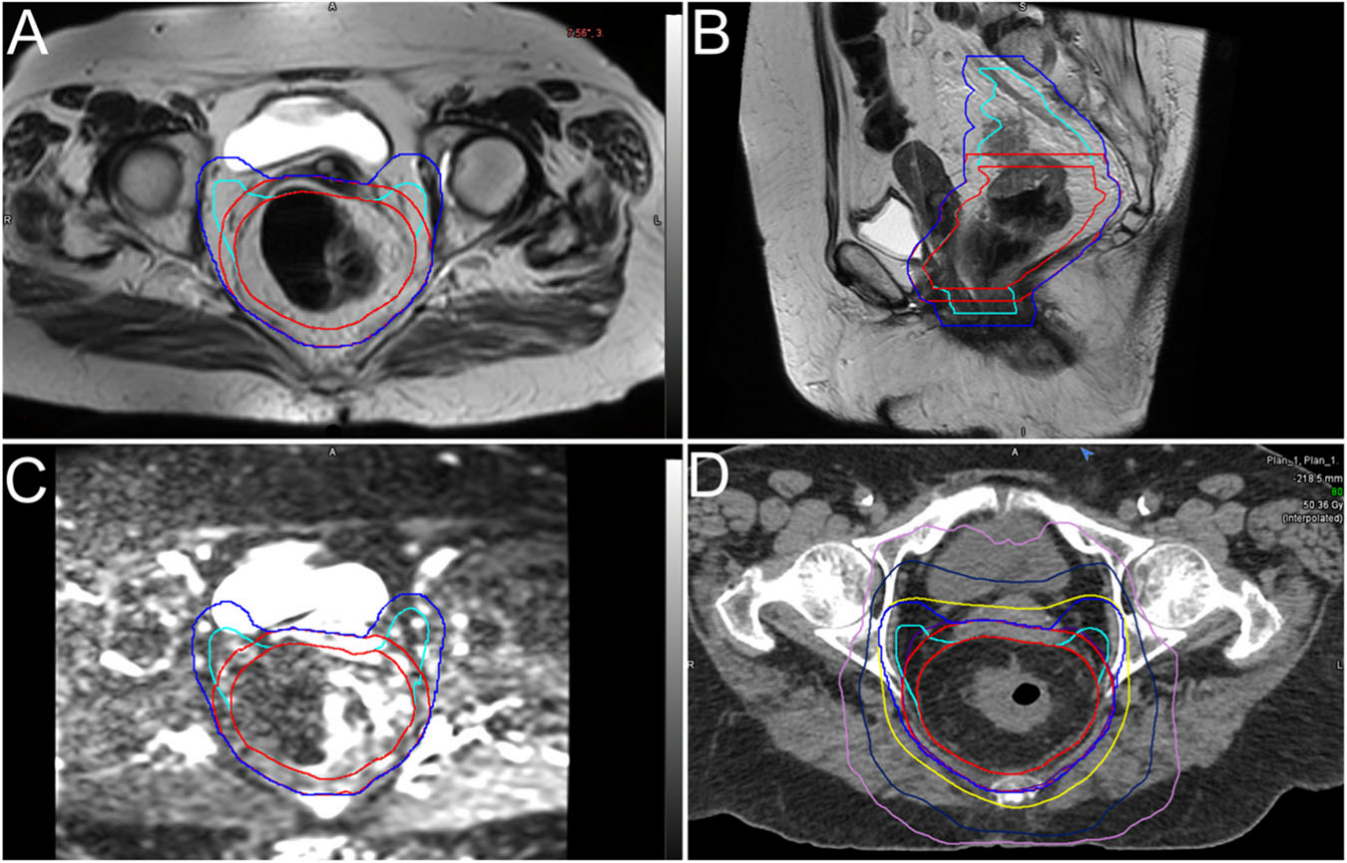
Axial T2- (A), sagittal T2w (B) images, b1000 DWI (C), and related centering CT (D) with superimposed GTV (red line), PTV (blue line) and distribution of doses in a patient with rectal cancer
